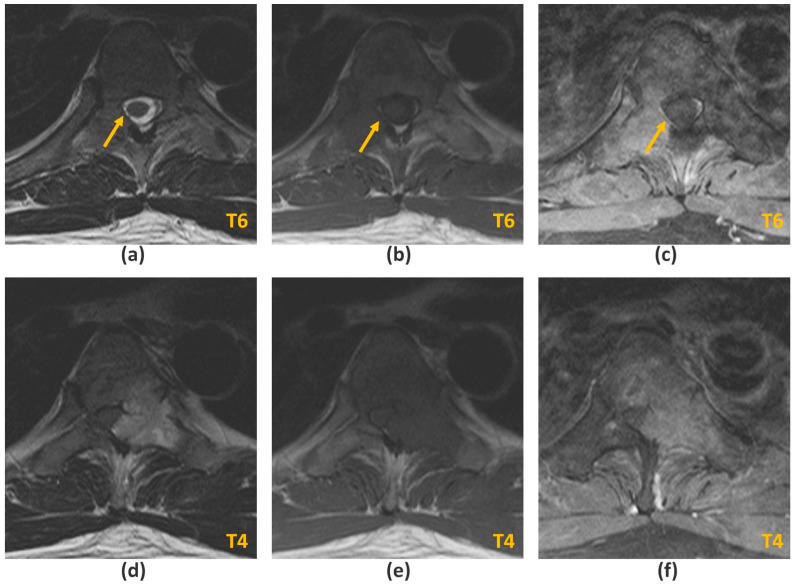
Axial T2-weighted (a), pre-contrast T1-weighted (b) and post-contrast fat-suppressed T1-weighted (c) MR images of a lung carcinoma patient with metastatic spinal cord compression (MSCC) at the level of T6. Epidural tumour extension is noted with thecal sac indentation but no spinal cord abutment (solid arrows), best demonstrated on the T2-weighted sequence (Bilsky 1b). Marrow infiltration by the tumour is indicated by hypointensity on the T1-weighted sequence, with corresponding enhancement seen on the post-contrast sequence. Axial T2-weighted (d), pre-contrast T1-weighted (e) and post-contrast fat-suppressed T1-weighted (f) MRI images of the same patient at a higher level (T4) show high-grade MSCC with complete obliteration of the CSF spaces, again best demonstrated on the T2-weighted sequence (Bilsky 3). Prominent enhancement of the epidural and vertebral component of the tumour is seen in post-contrast images.
